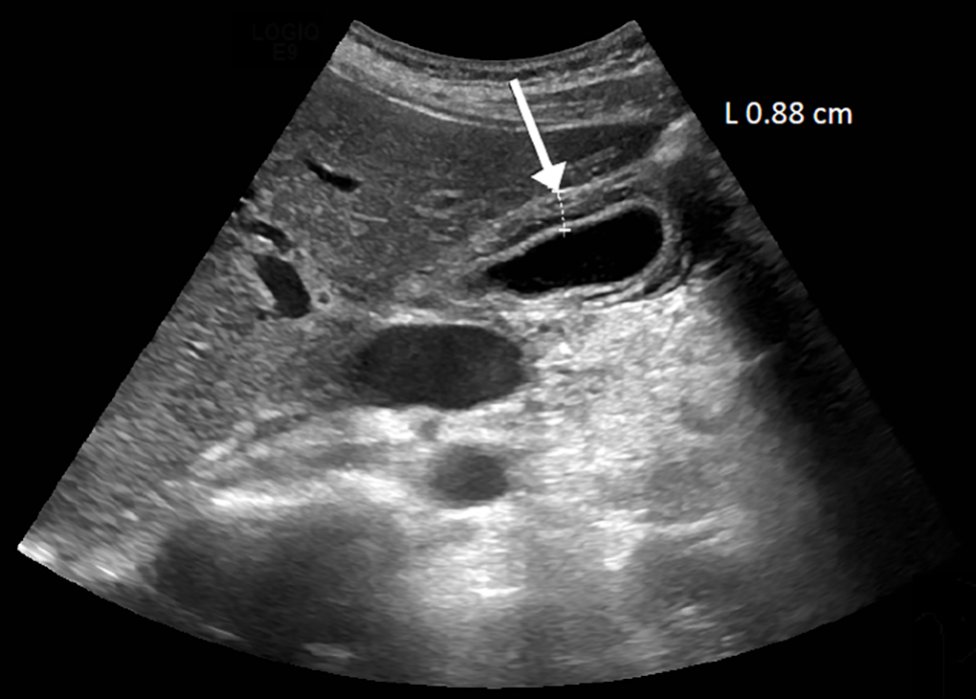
Abdominal ultrasound shows signs of an AAC with gall bladder wall thickening (white arrow). AAC, acute acalculous cholecystitis.
